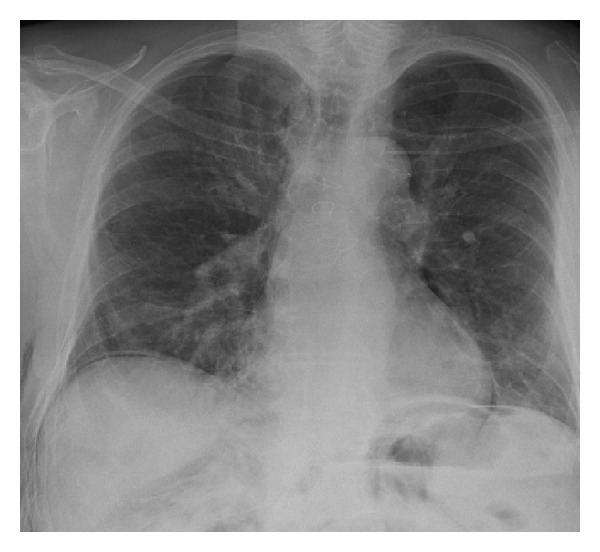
Intra-abdominal free air on an erect chest X-ray.
